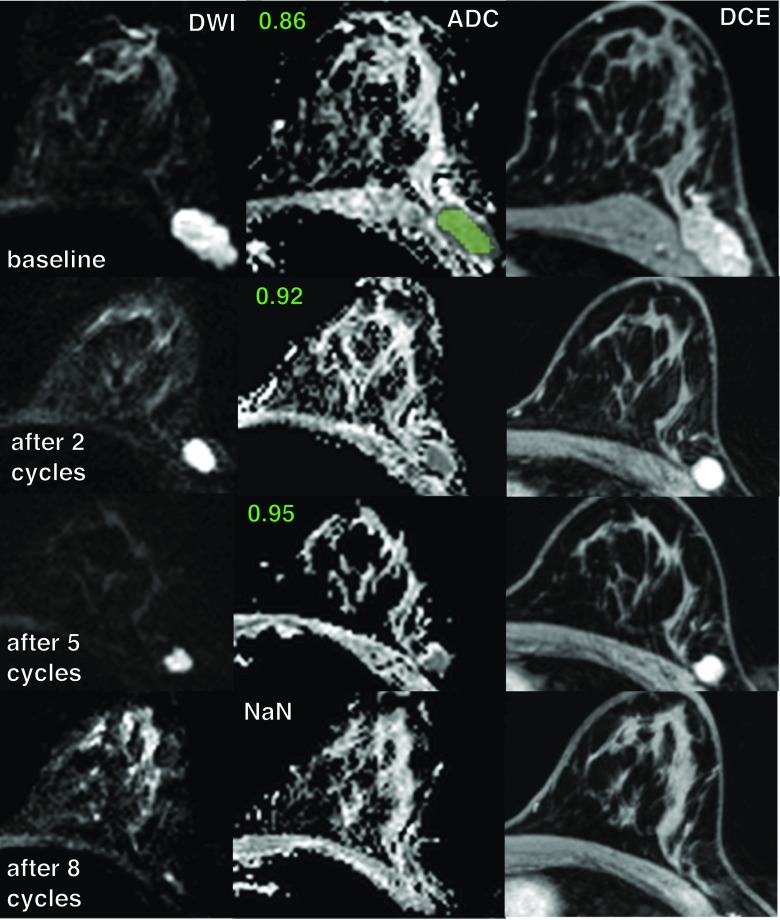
Examples of DWI and CE MRI in a 37-year-old patient with invasive ductal carcinoma and pathologic complete response to neoadjuvant chemotherapy. Mean ADC values (×10^-3^ mm^2^/s) measured before and during the therapy are depicted next to the corresponding ADC maps. An example of a segmented region of interest fills the lesion (green colour) in the baseline ADC map. *NaN* value missing
